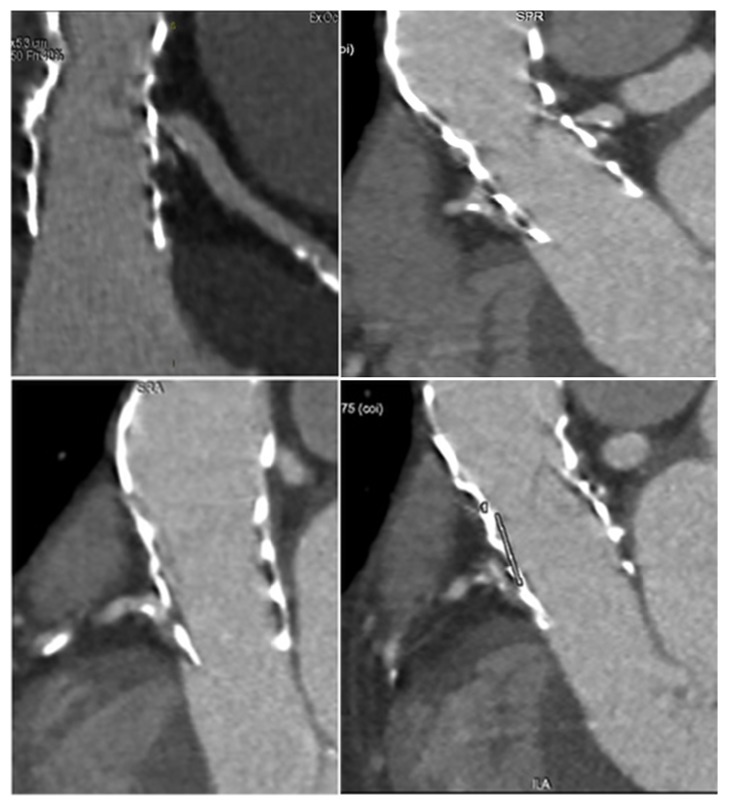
Follow-up gated CT scan demonstrating bioprosthesis malposition with respect to right and left coronary.
